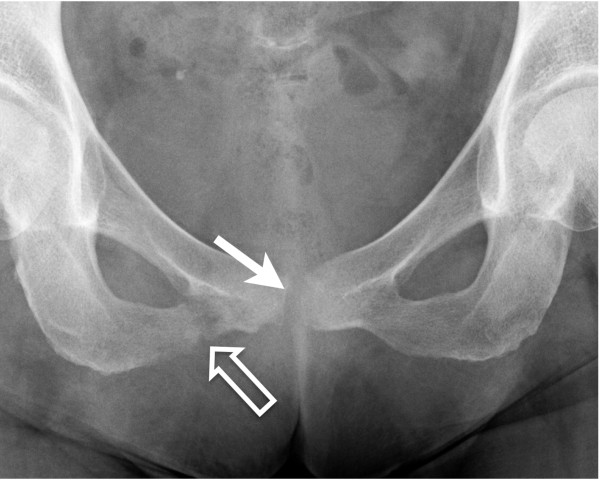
Anteroposterior radiograph of the pelvis shows a fracture of the inferior pubis ramus (open arrow) and irregularity of the symphisis pubis (arrow).
